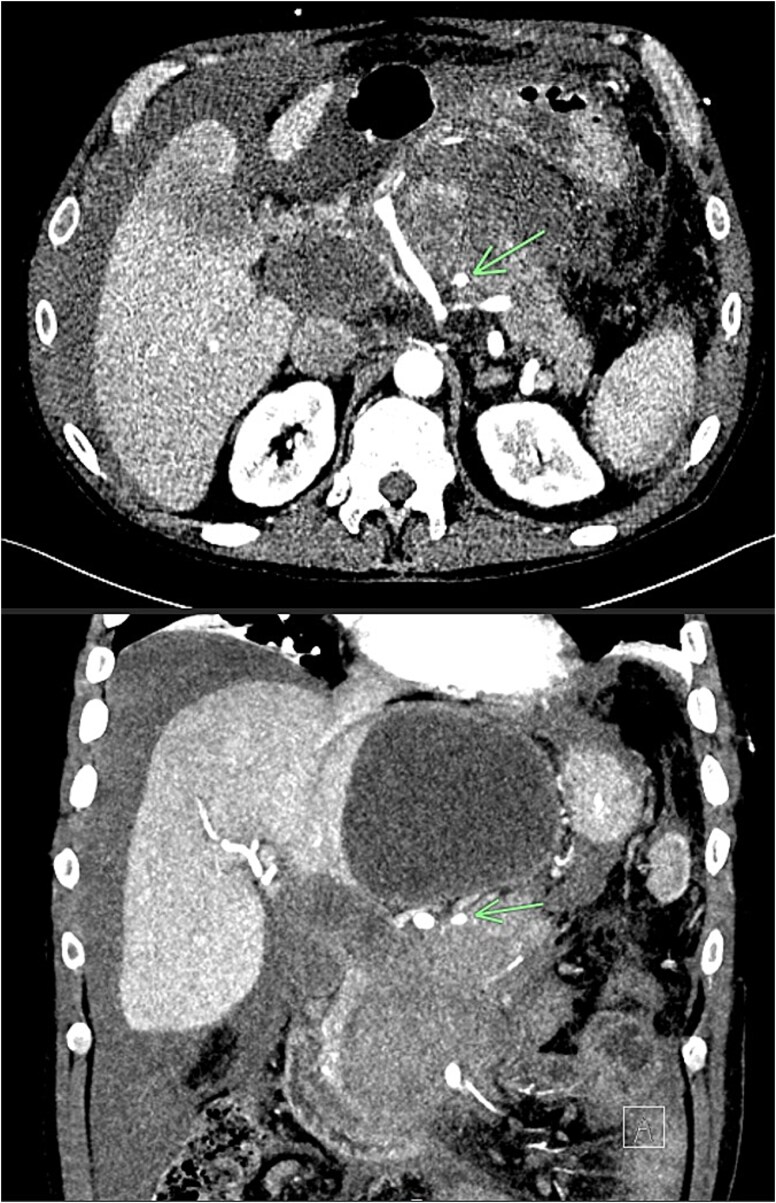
CT angiogram of abdomen axial and coronal view; small pseudoaneurysm arises from a branch of the SMA (arrow). Large haematoma in the head of the pancreas and diffuse haemoperitoneum throughout the abdomen/pelvis.
